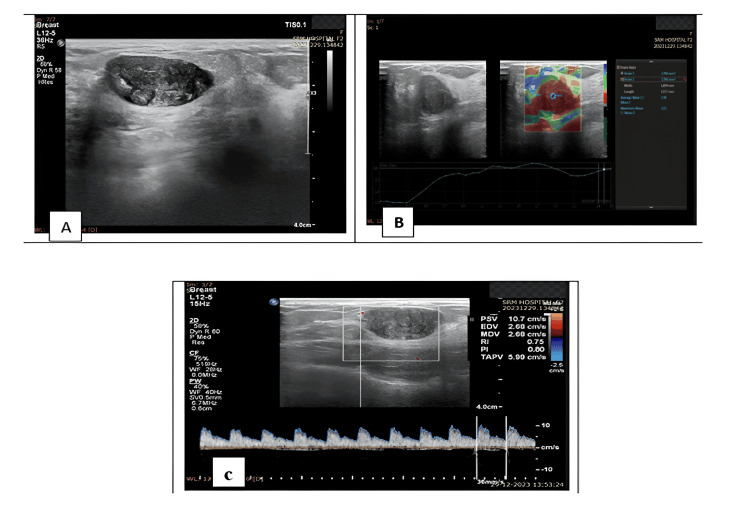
A 28-year-old female patient presented with a right breast lump A: Greyscale ultrasound showed a well-defined, wider-than-taller, oval-shaped hypoechoic lesion in the right upper outer quadrant. B: Strain elastography measured a strain ratio of 2.48. C: Doppler showed a PI of 0.8 and a RI of 0.75. FNAC confirmed the lesion as a fibroadenoma. PI: pulsatility index; RI: resistance index; FNAC: fine needle aspiration cytology.
